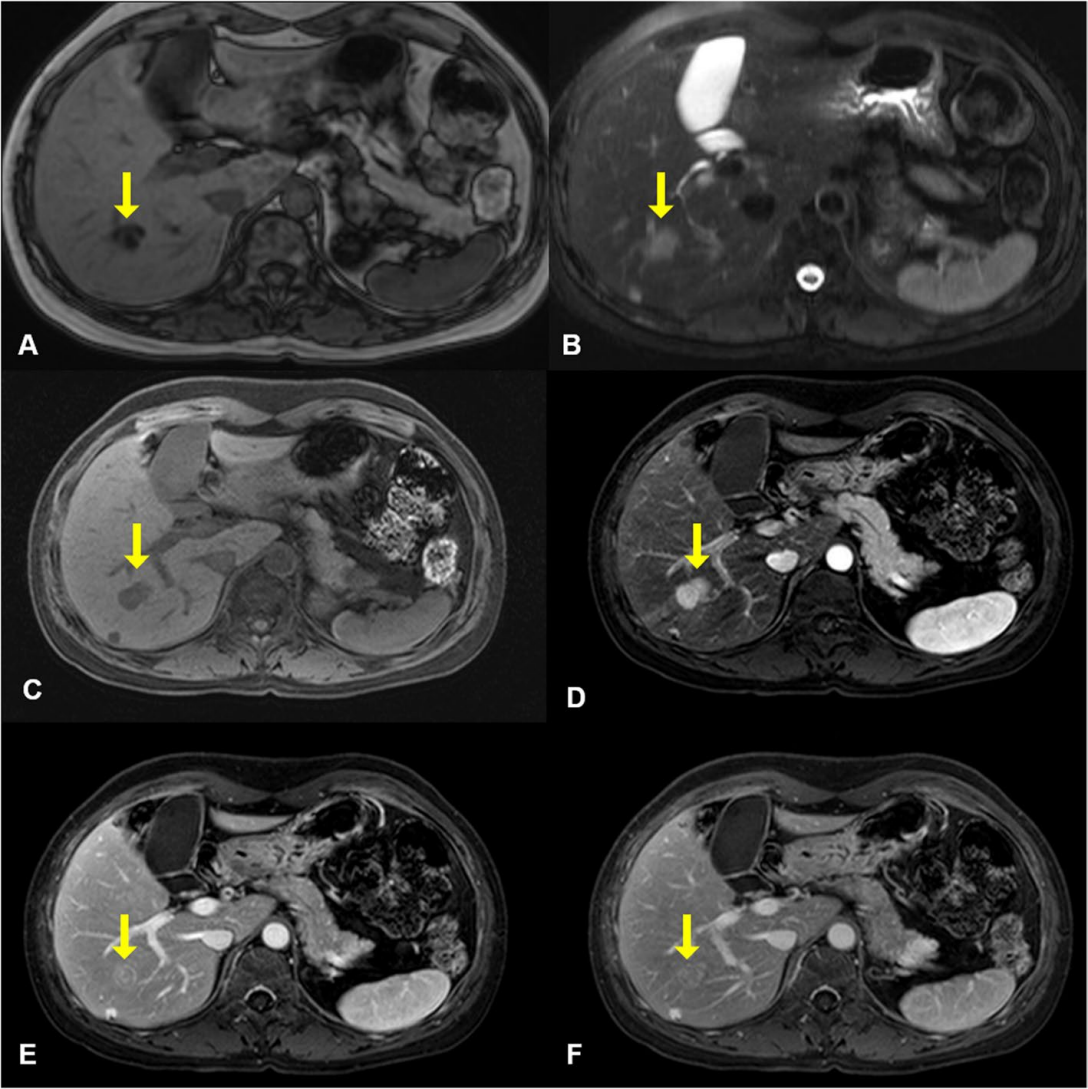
Abdominal magnetic resonance images of the liver mass. The mass in the right hepatic lobe demonstrated low signal intensity on T1‑weighted images (A) and intermediate signal intensity on T2‑weighted images (B). Dynamic gadoxetic acid-enhanced magnetic resonance images of the liver mass showed low signal intensity on pre-contrast image (C), intense enhancement on the arterial phase image (D), and equivocal washout of the tumor on portal phase (E) and delayed phase (F) images. The yellow arrow indicates the mass
